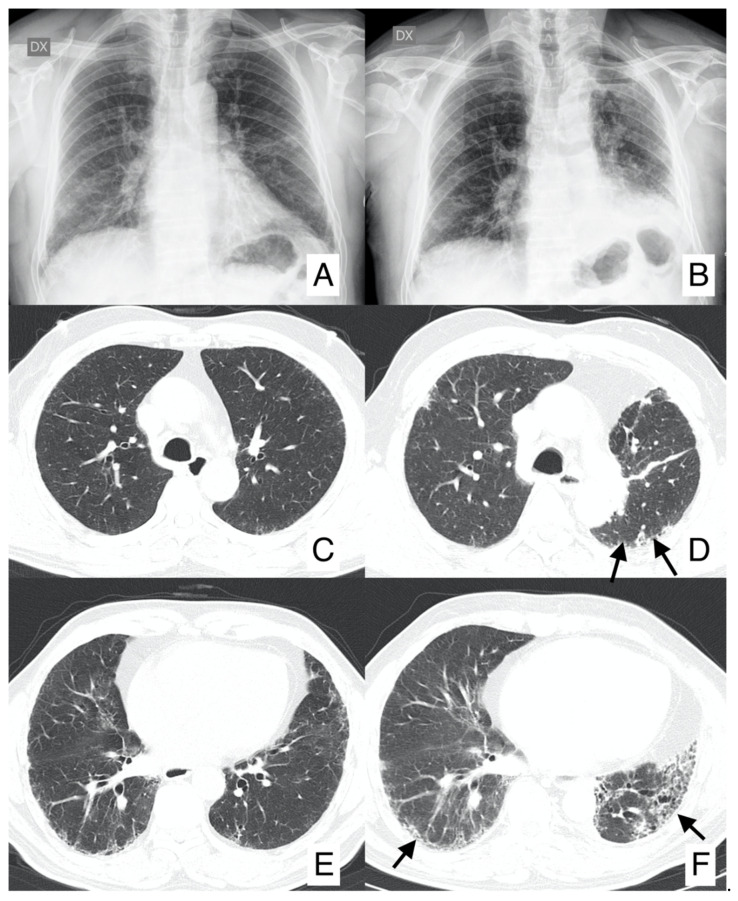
Progression of IPF disease after surgical resection of nodular neoplastic lesion (same patient as Figure 3, having IPF complicated by squamous cell carcinoma). Chest radiographs (A,B) acquired before and after surgical resection: it is possible to appreciate the increased reticular pattern (namely in the left lung). Axial CT images before (C,E) and after surgery (D,F). CT images clearly show progression of reticulations—well-depicted in subpleural regions (black arrows).
